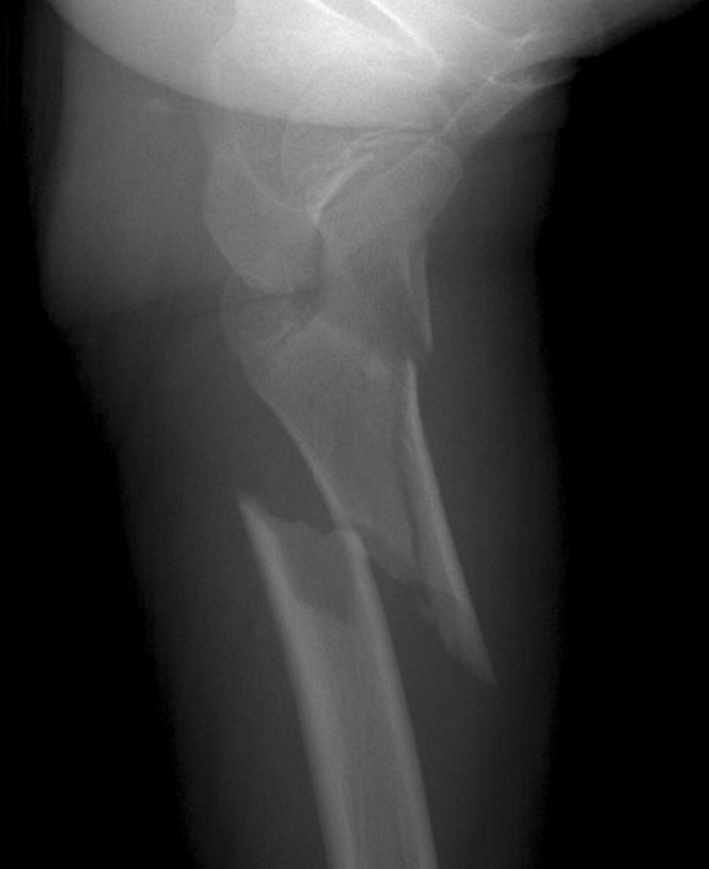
Eight-year-old girl: lateral radiographs reveal a cervicotrochanteric fracture (type III in Delbet’s classification; AO classification type II) with a subtrochanteric fracture of the left femur
